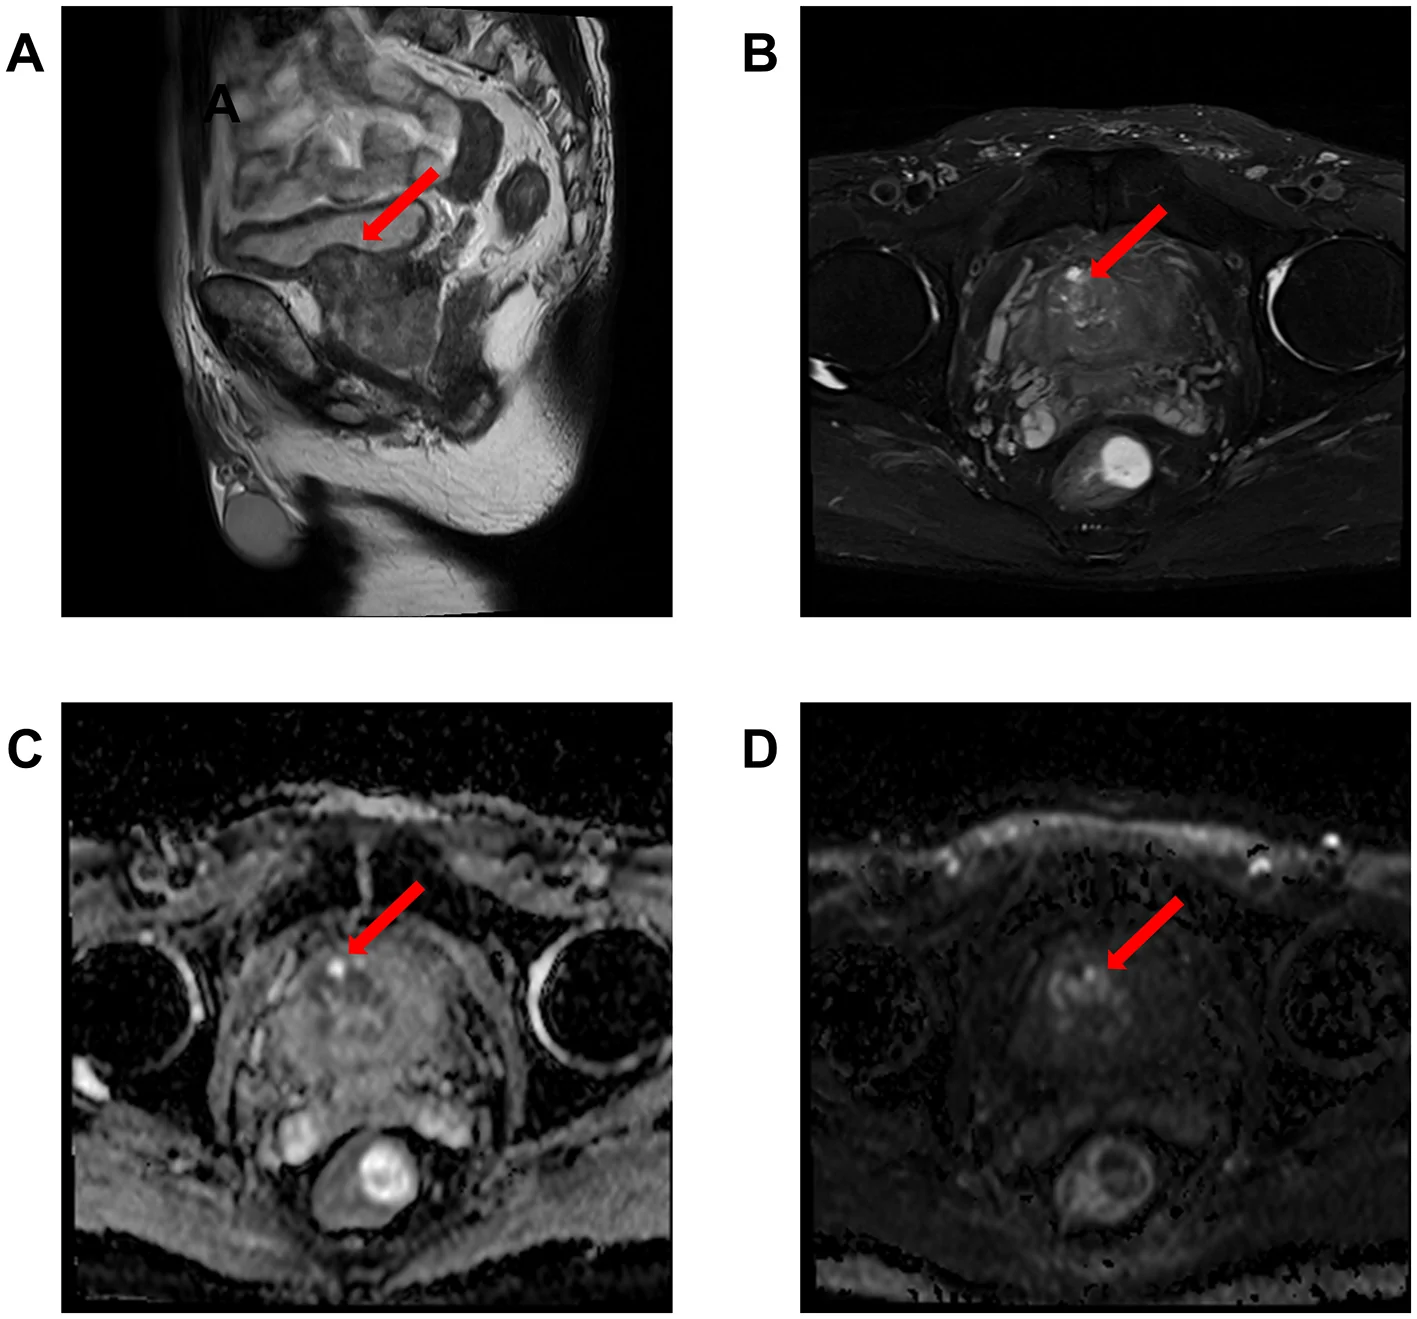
(A) Prostate protruding into the bladder. (B) Prostate MRI scan T2WI showed low signal. (C) Prostate MRI scan ADC showed low signal. (D) Prostate MRI scan DWI revealed hyperintense signal.
